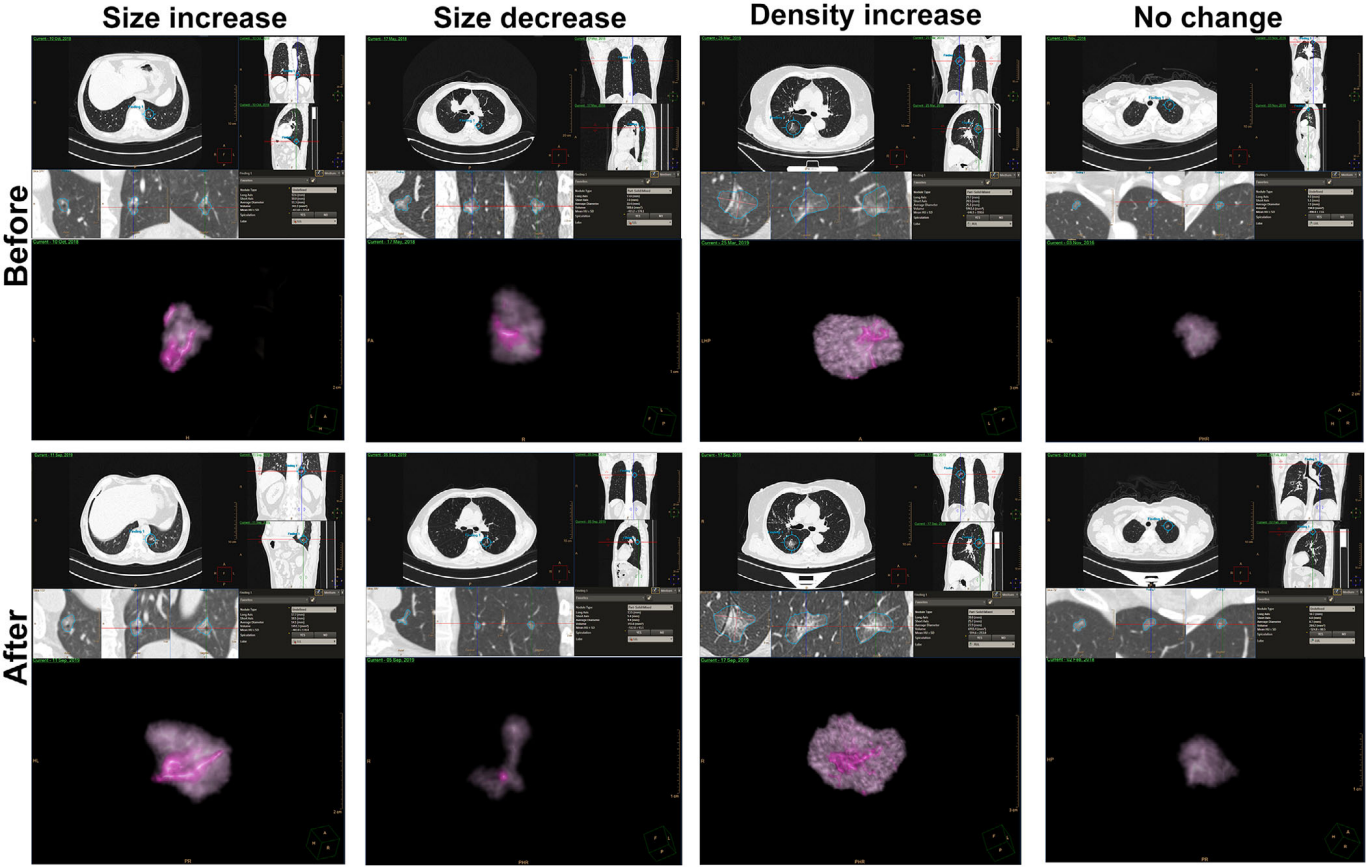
The representative images of four types of GGNs changes after treatment, including size increase, size decrease, density increase (solid component increase or development of a new solid component), and no change. For the volume rendering images, dark gray mass represents the shape and volume of delineated GGN on the CT images, and pink shape represents the vessels
